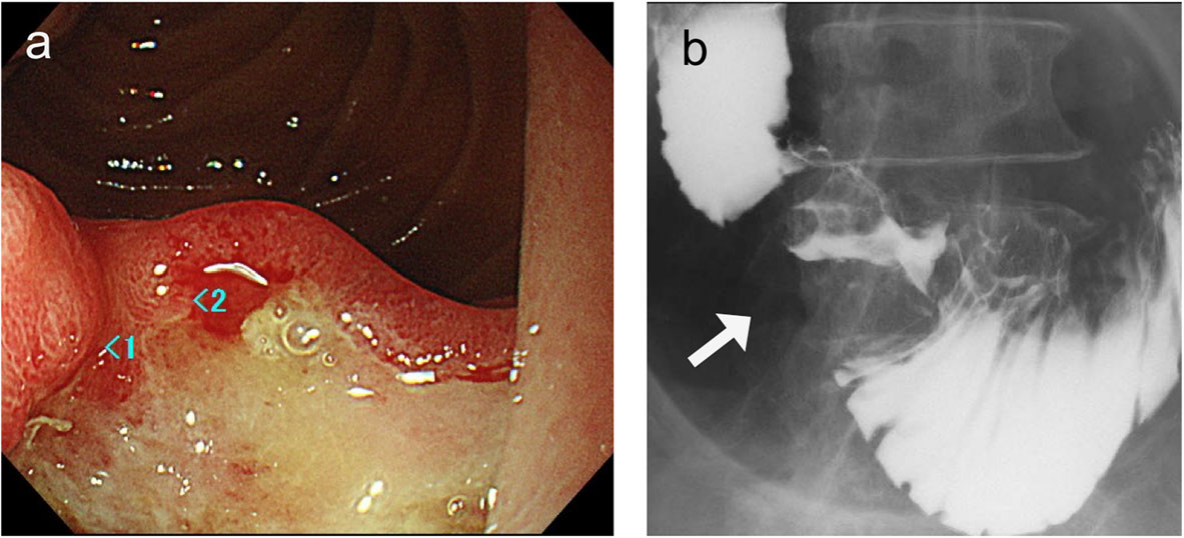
a Upper gastrointestinal endoscopy revealed a large, hemorrhagic, ulcerative tumor with an irregular border in the second portion of the duodenum. b X-ray radiography showed an ulcerative tumor 4.5 cm in diameter with an irregular border
